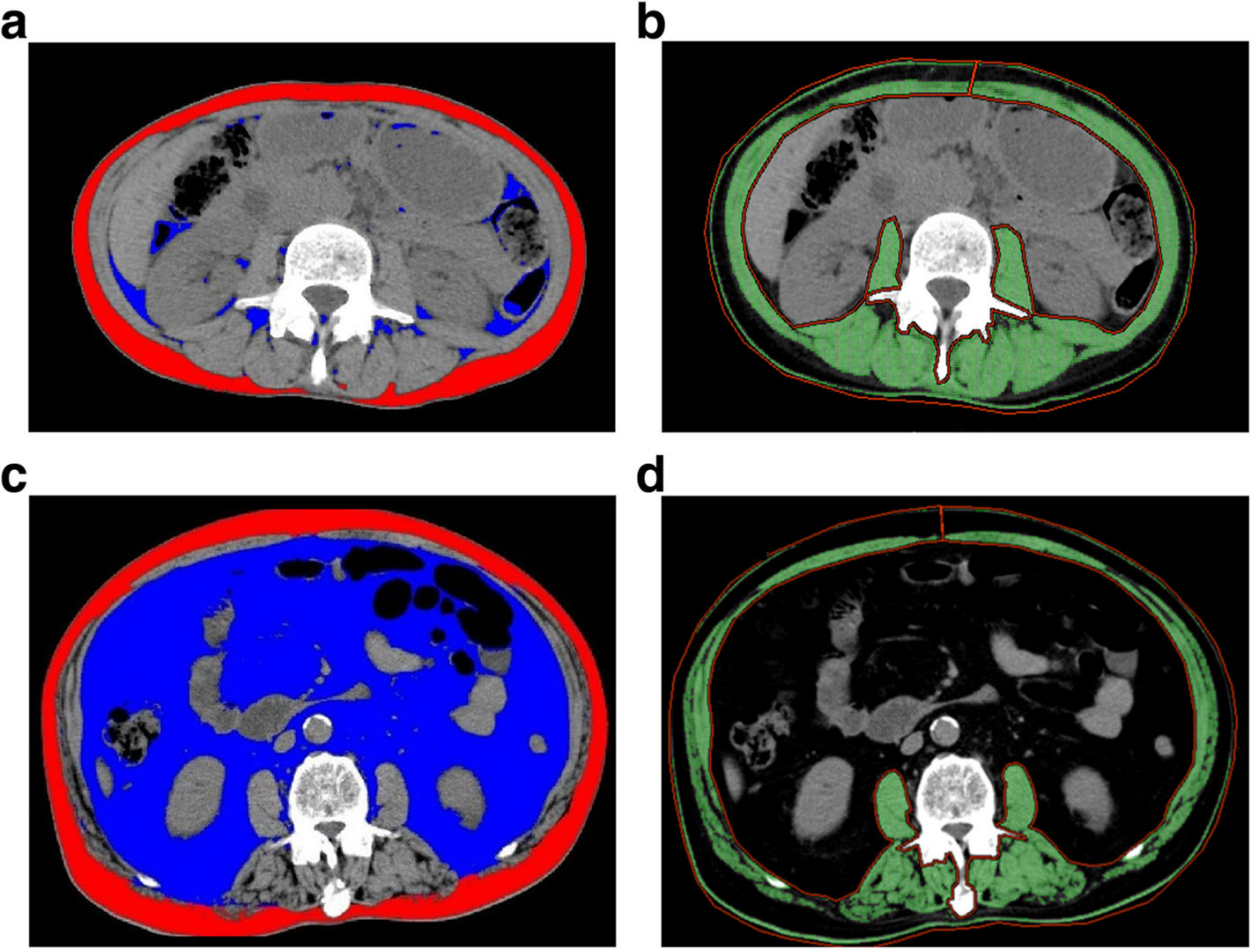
Computed tomography (CT) images at the third lumbar vertebra level. The visceral adipose tissue area (VATA) is highlighted in blue, while the subcutaneous adipose tissue area (SATA) is highlighted in red. Areas of total skeletal muscle is highlighted in green. a, b CT image of a patient with low VATA/skeletal muscle index (SMI; VATA, 8.0 cm^2^/m^2^; SATA, 43.8 cm^2^/m^2^; SMI, 40.5 cm^2^/m^2^; VATA/SMI, 0.20). c, d CT image of a patient with high VATA/SMI (VATA, 359.0 cm^2^/m^2^; SATA, 125.2 cm^2^/m^2^; SMI, 42.5 cm^2^/m^2^; VATA/SMI, 8.45)
